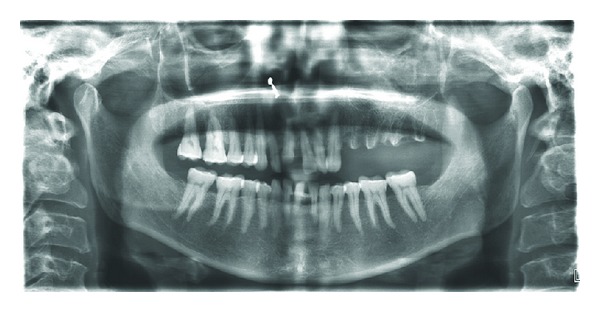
Orthopantomogram reveals missing teeth (24, 25, 26, 27,  and  28) with unhealed sockets.
